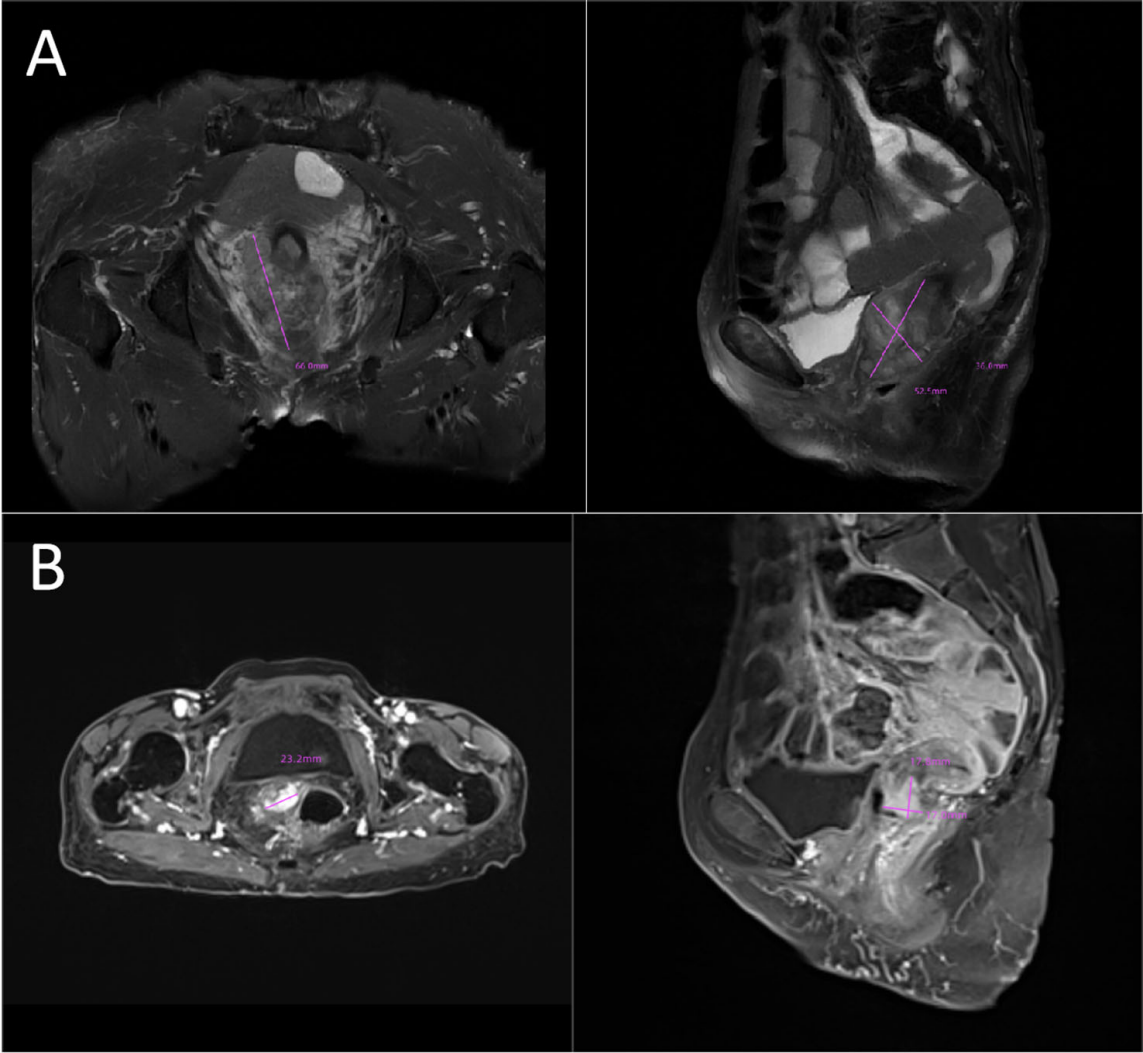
(A) Initial tumor mass:MRI image showing an occupying lesion in the anterior lip of the cervix, which is considered to be sarcomatoid carcinoma of the cervix (66mmx52.5mmx36mm). The boundary with the anterior wall of the rectum and the posterior wall of the bladder is unclear (FIGO stage IVA). (B) Post-treatment shrinkage:The pelvic MRI results showed a significant reduction in the size of the patient’s tumor (23.2mmx17.8mmx17.0mm). MRI, magnetic resonance imaging.
